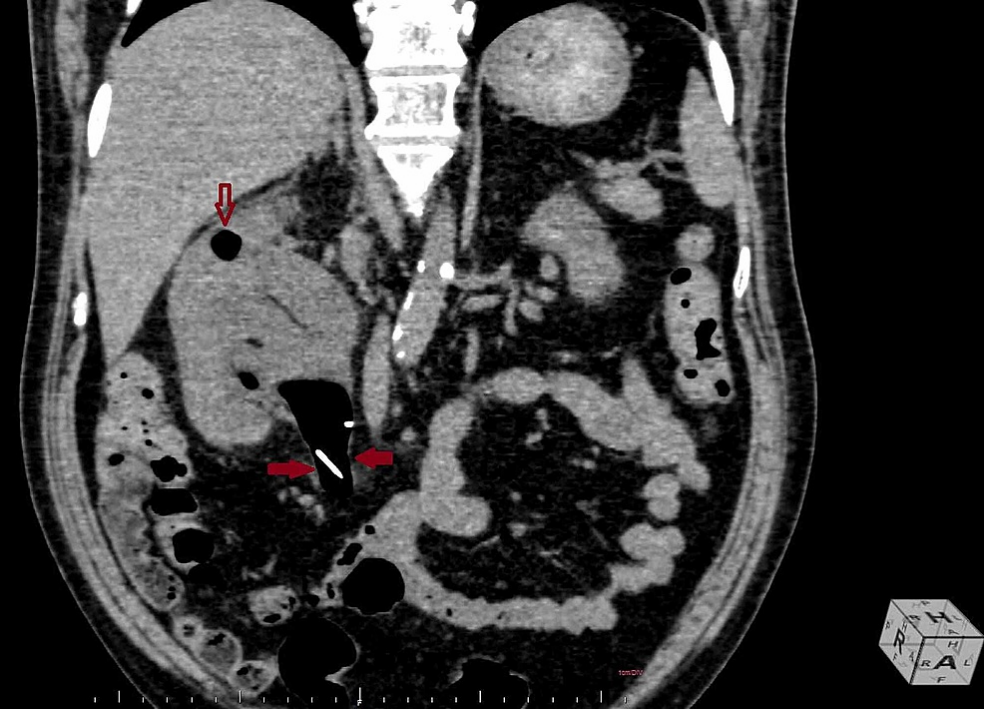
Sagittal reconstruction of CT abdomen revealed a massive air pocket in the pelvis of right kidney, extending into the upper ureter (red arrows). The proximal end of the DJ stent was noted in the upper ureter, suggestive of migrated DJ stent. A small air pocket was noted in the upper calyx, indenting into the renal cortex (empty red arrow).
